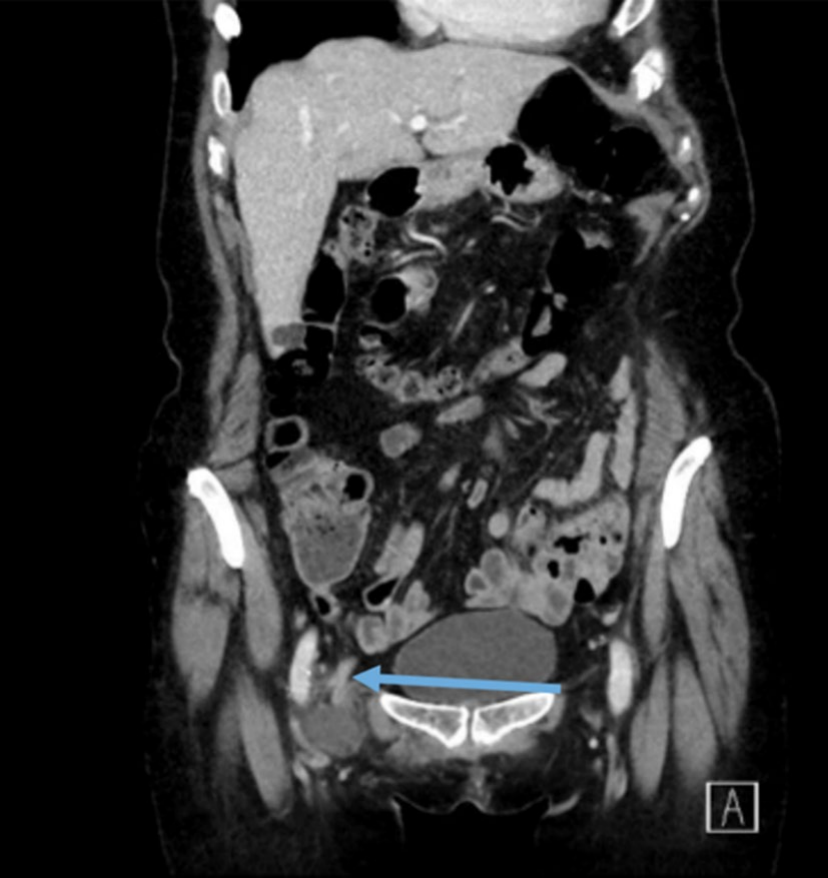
The Blue arrow shows the appendix going into the femoral hernia on the CT
